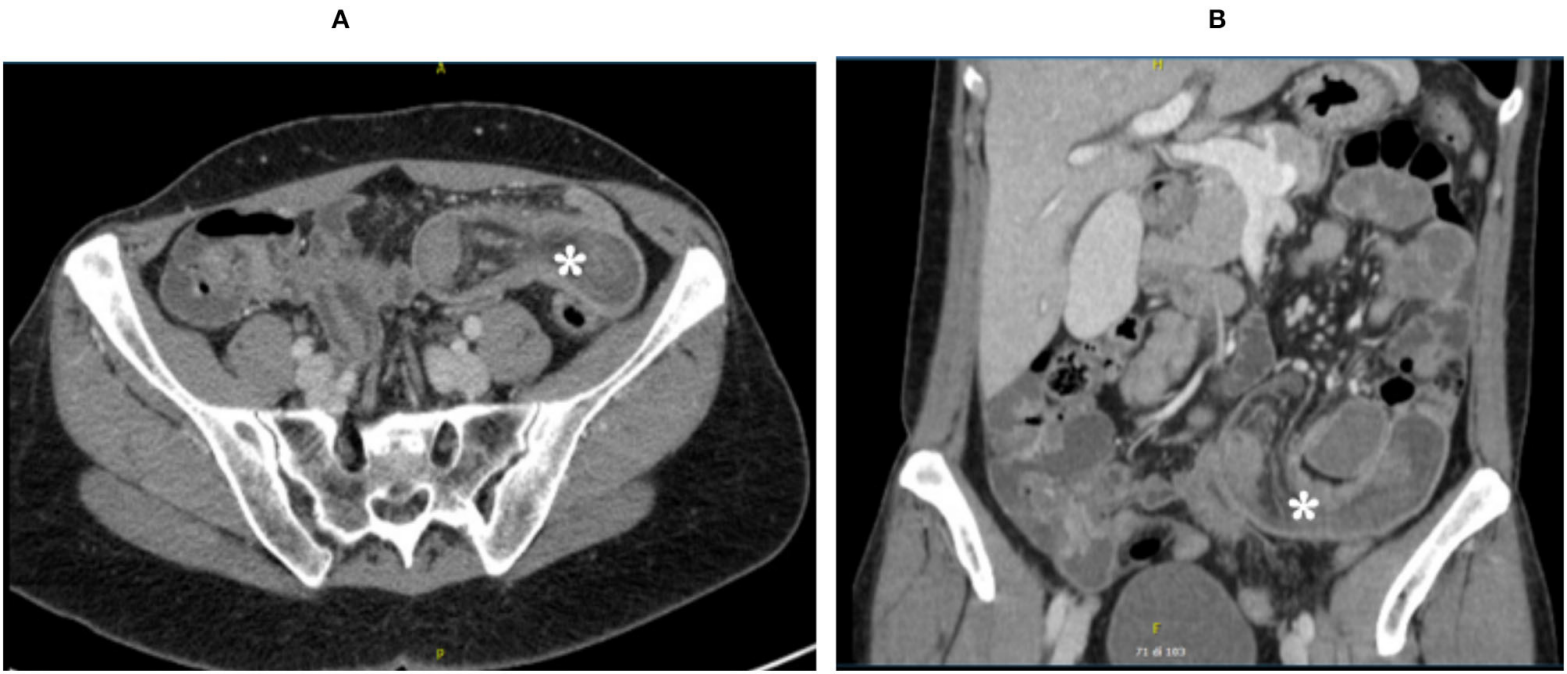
Abdominal CT scan: *indicates the ileo-ileal intussusception. (A) CT transverse section. (B) CT coronal section.
